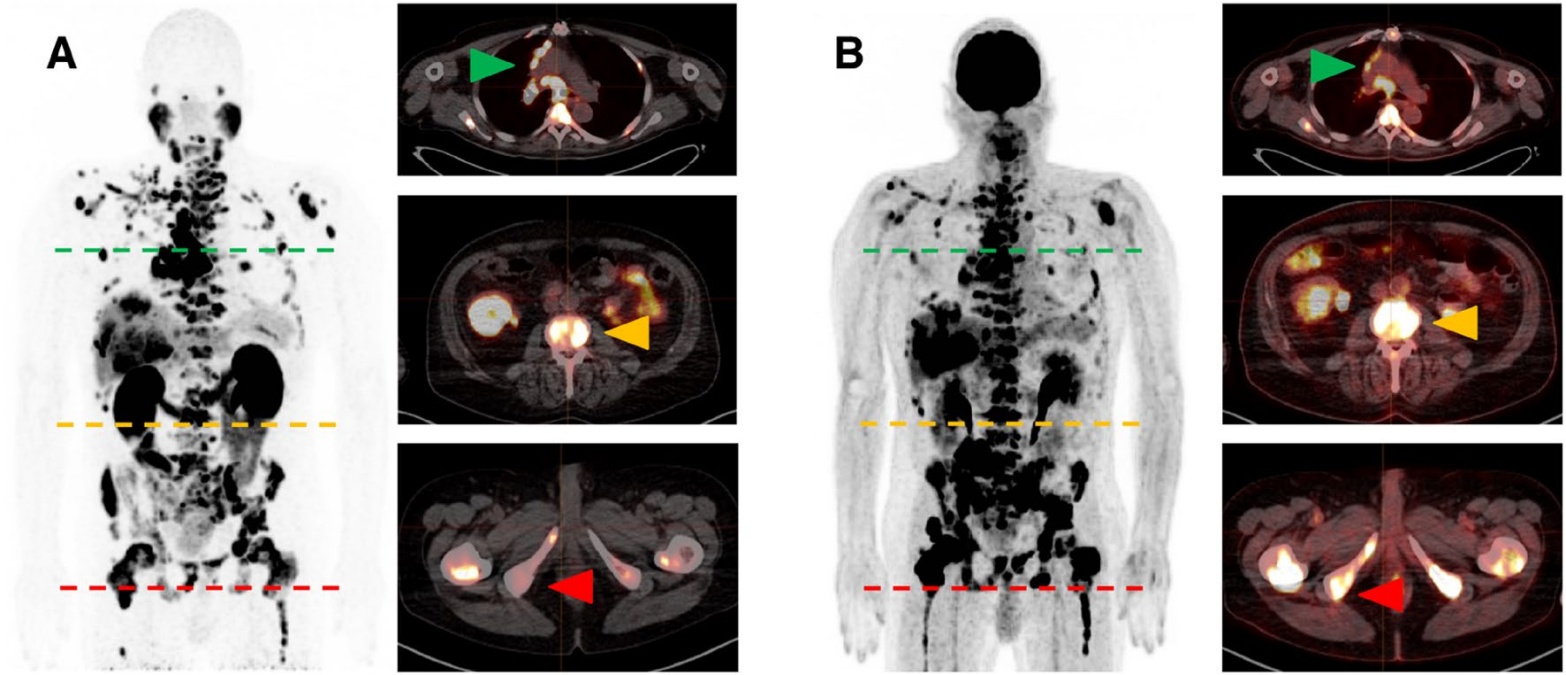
Representative whole-body and transversal slice images from (A) baseline [^68^Ga]Ga-PSMA-11 PET/CT scan and (B) baseline [^18^F]FDG-PET/CT scan of a mCRPC patient showing different patterns of [^68^Ga]Ga-PSMA-11 uptake and [^18^F]FDG uptake in different lesions. Green arrows denote lymph node metastases with intense [^68^Ga]Ga-PSMA-11 and moderate [^18^F]FDG uptake. Yellow arrows indicate bone metastases with intense uptake of both radiotracers. Red arrows indicate bone metastases with faint [^68^Ga]Ga-PSMA-11 uptake but intense [^18^F]FDG uptake.
